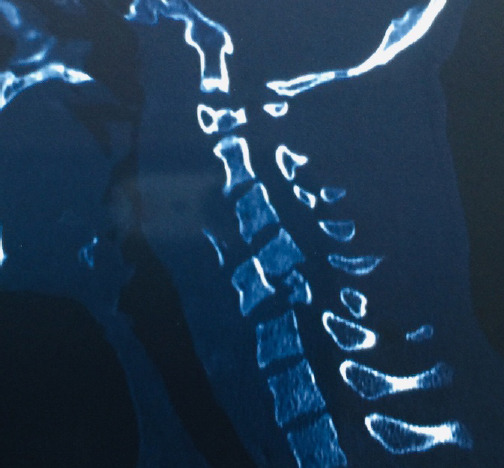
Computed tomography sagittal section showing burst fracture of C5 with spondylolisthesis of C4 and spondyloptosis of C6.
